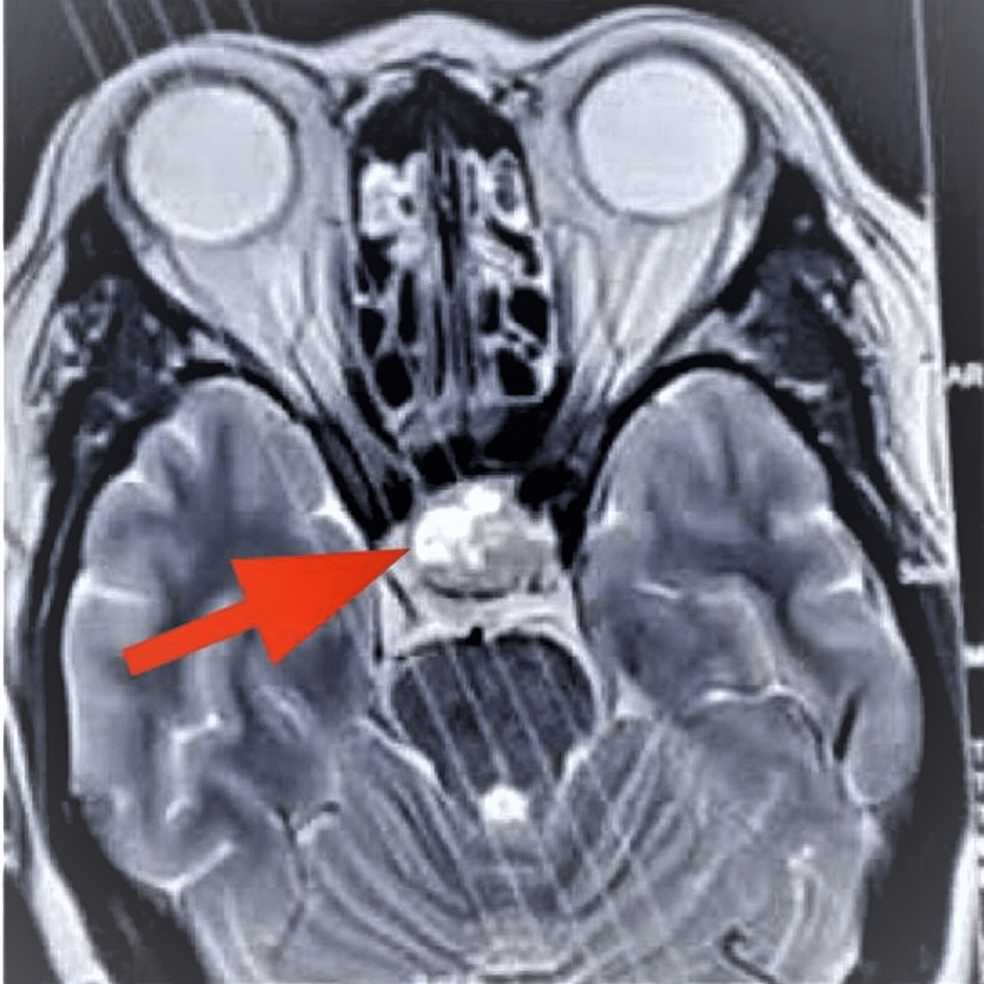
MRI of brain showing pituitary mass
